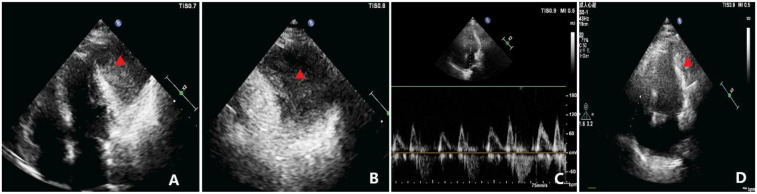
Echocardiographic findings. (A) Apical four-chamber view showing a homogenous mass, measuring approximately 69 × 50 mm, adjacent to the anterolateral wall of the left ventricle (indicated by the triangle), seemingly within the pericardial cavity. The mass is well-circumscribed, and the left ventricular anterolateral wall has mild hypokinesis. (B) Magnified view of (A) showing the relationship between the mass and adjacent structures in greater detail (indicated by the triangle). (C) Diastolic flow velocities across the mitral and tricuspid valves demonstrating significant respiratory variation, with the change in peak diastolic velocity during inspiration exceeding 30%. (D) Postoperative TTE apical view showing marked reduction in the residual mass burden compared with baseline (indicated by the triangle), with improvement in regional wall motion.
